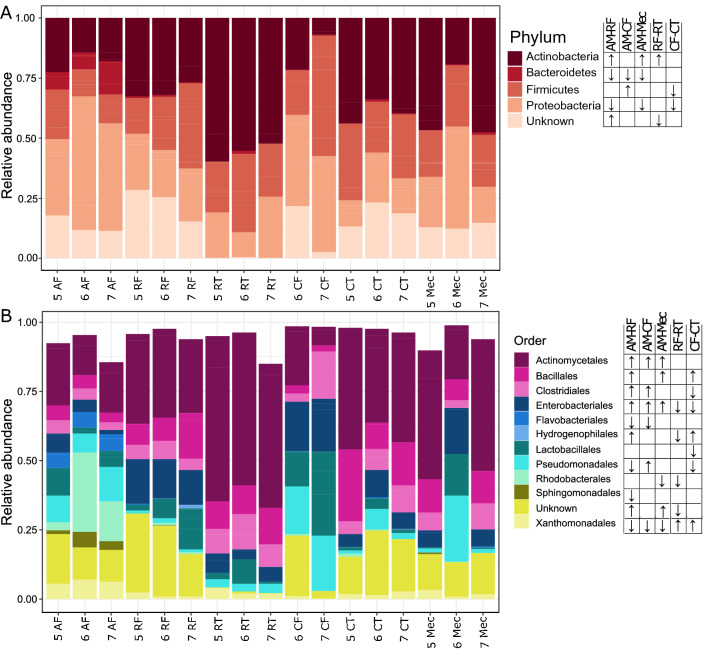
Mean relative abundance of bacterial phylum level (A) and order level (B) ESVs in each sample type (GIT component and amniotic fluid) at each gestational age (5, 6 and 7 months). Significant phylum-level and order-level changes in relative abundance between sample types are denoted next to the taxonomic key. For each pair of sample types, the arrow indicates the direction of change in abundance in the second sample type relative to the first. ESVs are clustered by colour to the levels of phylum and order. ESVs with taxonomic assignments at the phylum level and order level with confidence values lower than 90% are denoted as ‘Unknown’. Levels of gDNA in caecal fluid from foetuses at 5 months gestation were below the concentration threshold (0.5 ng/µL) for samples to be included in the sequencing run. Amniotic fluid (AF), ruminal fluid (RF), ruminal tissue (RT), caecal fluid (CF), caecal tissue (CT), meconium (Mec).
